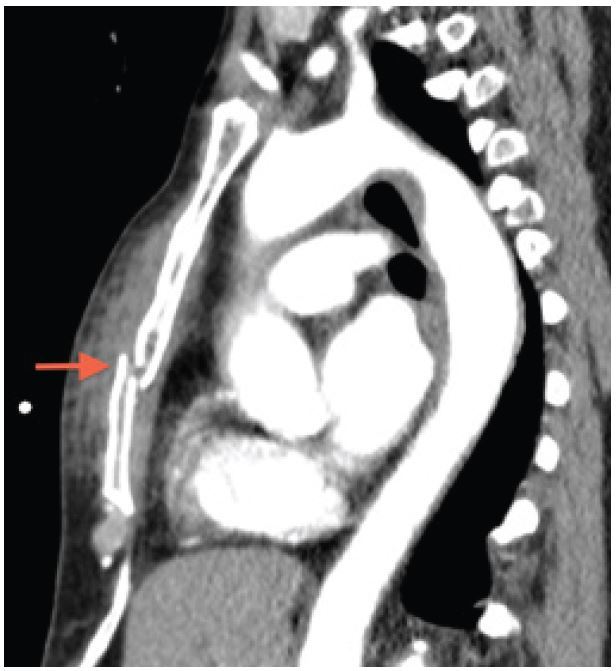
Computed tomography identifying the same displaced sternal fracture.
